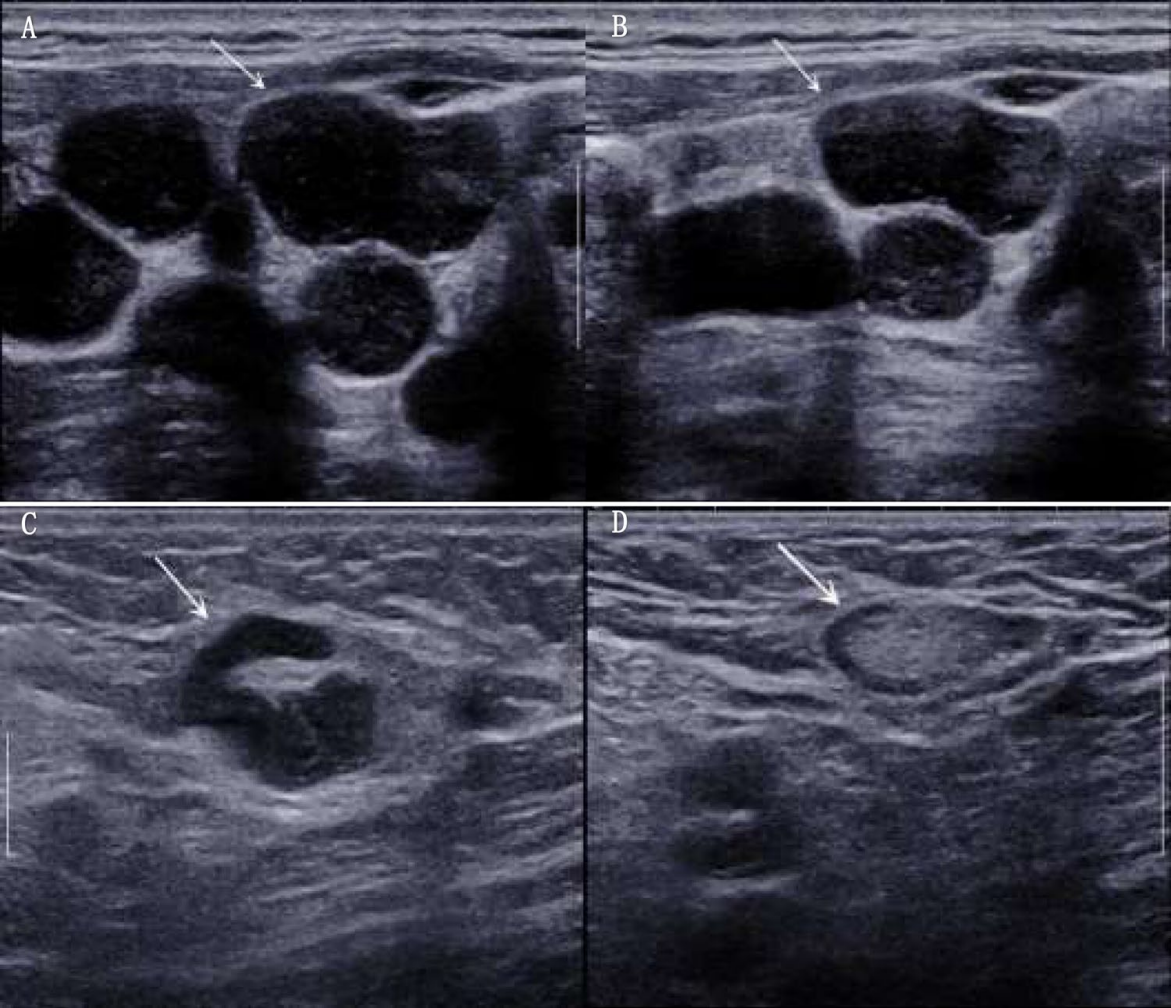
Ultrasound manifestations of the lymphoma. The image on the left is an ultrasound image of the left cervical lymph node in a patient with DLBCL before chemotherapy, showing the loss of lymphatic hilum structures (A). On the right side is an image of the lymph node in the same area of the same patient after standardized treatment (B). It can be seen that lymphoma with hilum loss is not effective with chemotherapy. However, lymphomas that showed the presence of lymphatic hilum structures on ultrasound decreased significantly in size after standardized chemotherapy, and the morphology of the lymph nodes gradually normalized (C,D).
